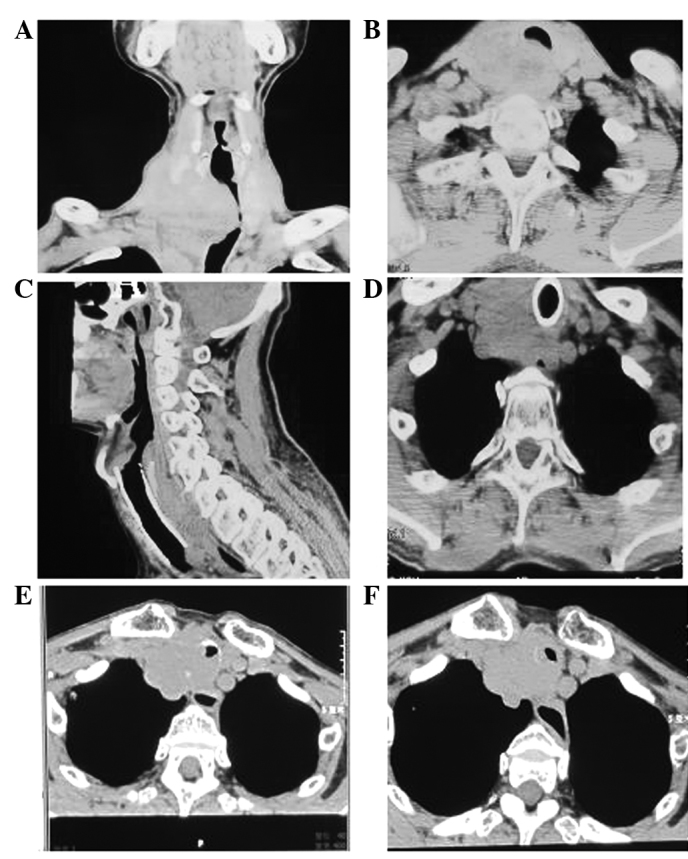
A 69-year-old male patient presented with esophageal cancer with post-operative cervical lymph node metastasis (LNM) compressing and infiltrating the trachea causing severe dyspnea. (A and B) Coronal and axial computed tomography (CT) scan showing LNM compressing and infiltrating the trachea. (C and D) Sagittal and axial CT scan 2 days after placement of the stent showing that the stent was fully expanded against the tumor, with a clear airway. (E and F) Two months after stent placement, regrowth of tumor tissue occurred in the lower end of the stent, causing dyspnea again.
